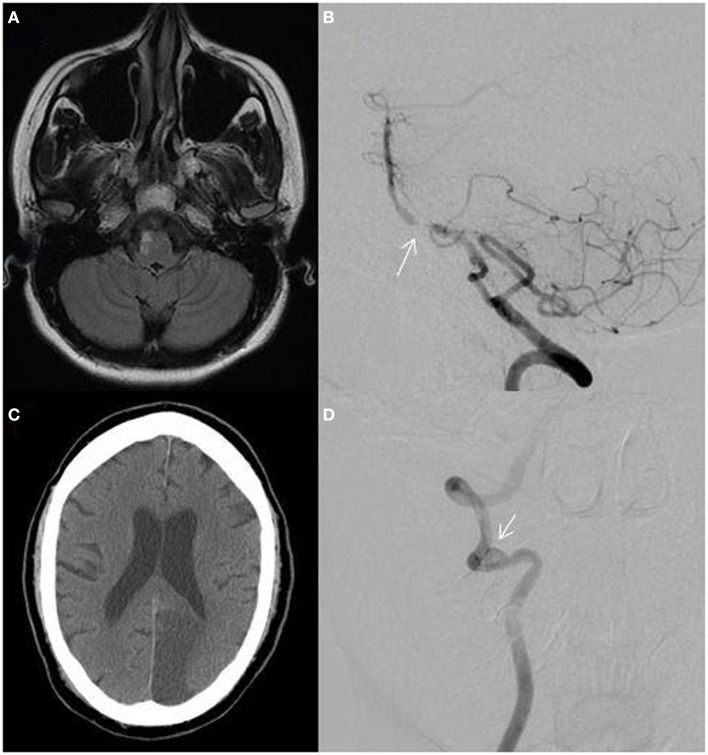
(A) MRI fluid-attenuated inversion recovery (FLAIR) sequence showing a right lateral medullary infarction in a 32-year-old woman with a hypoplastic right vertebral artery shown in Figure 2A; (B) catheter angiogram of a 55-year-old African-American man showing atherostenosis at the vertebrobasilar junction (arrow); (C) non-contrast CT showing a left posterior cerebral artery territory infarction in a 60-year-old man with atrial fibrillation; (D) catheter angiogram showing a right distal vertebral dissecting aneurysm with intraluminal thrombus (arrow) in a 19-year-old man presenting with vertigo, ataxia, and a right cerebellar infarction.
